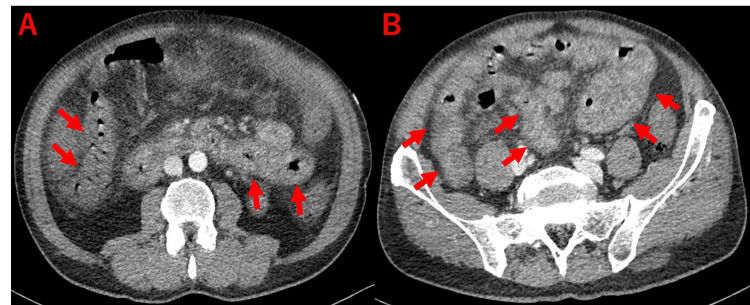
Contrast-enhanced CT scans The arrows demonstrate intestinal edema involving the small to large intestines CT: computed tomography
